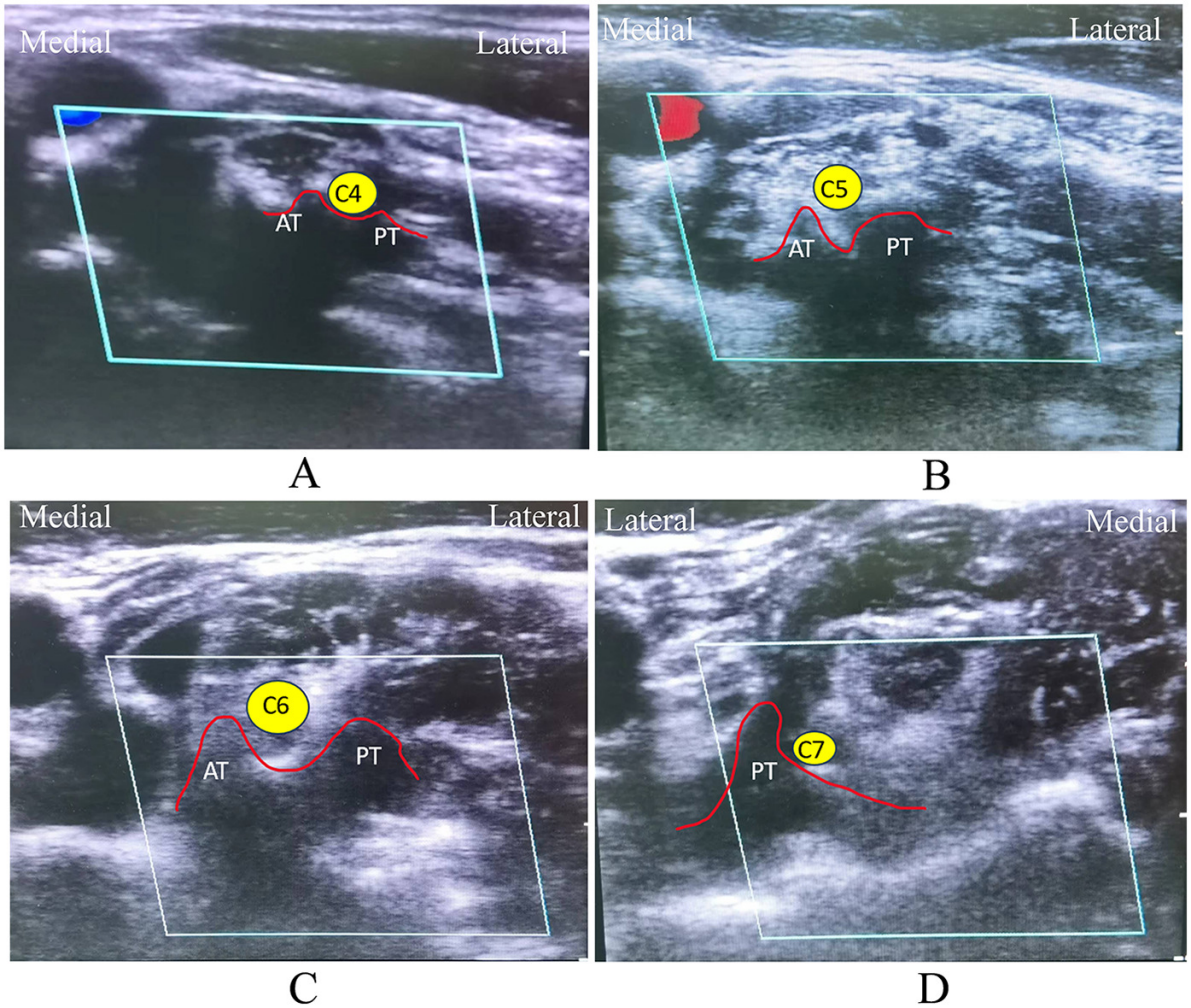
Ultrasound identification of cervical nerve roots. (A) Characteristics of horizontal nodules of C4 nerve root: there were two nodules in front and back under ultrasound, and the internodal sulci was shallow. (B) Characteristics of horizontal nodules of C5 nerve roots: there were two nodules in front and back under ultrasound, and the internodal sulci was deep. (C) Characteristics of horizontal nodules of C6 nerve roots: there were two nodules in front and back under ultrasound, and the anterior nodules of C6 protruded more than the posterior nodules. (D) Characteristics of C7 nerve root horizontal tubercle: Anterior transverse tubercle of C7 is underdeveloped, only posterior tubercle. C4: Fourth cervical nerve root, C5: fifth cervical nerve root, C6: sixth cervical nerve root, C7: seventh cervical nerve root. AT, anterior tubercle; PT, posterior tubercle.
